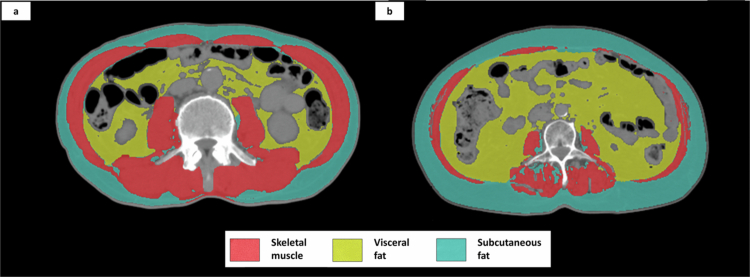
Examples of the distribution of skeletal muscle, visceral fat, and subcutaneous fat in the L3 section of abdominal CT in a) non-sarcopenia and b) sarcopenia cases.
